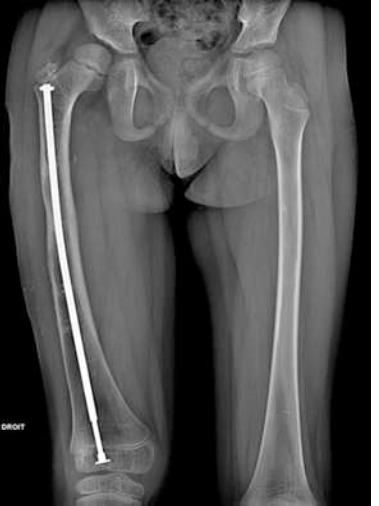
anteroposterior hip X-ray—reduced hip with no signs of avascular necrosis of the head at last follow-up
